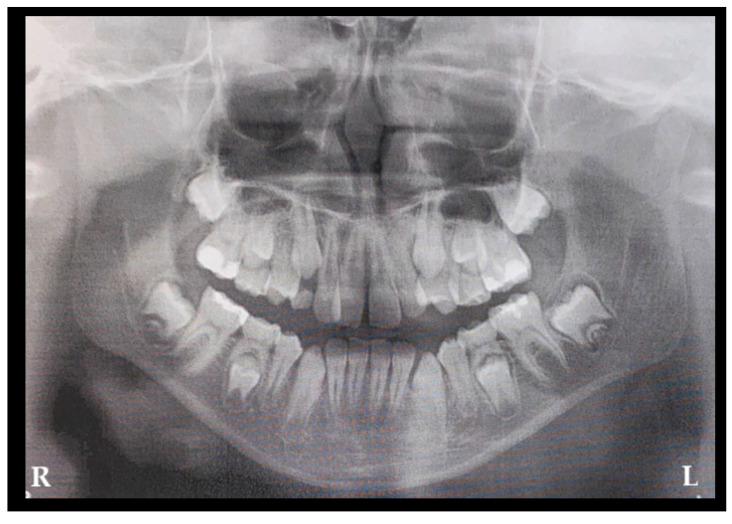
Initial scan—orthopantomogram (OPG) of a 13-year-old boy, consulted by a local orthodontist (abbreviations: R—right; L—left).
